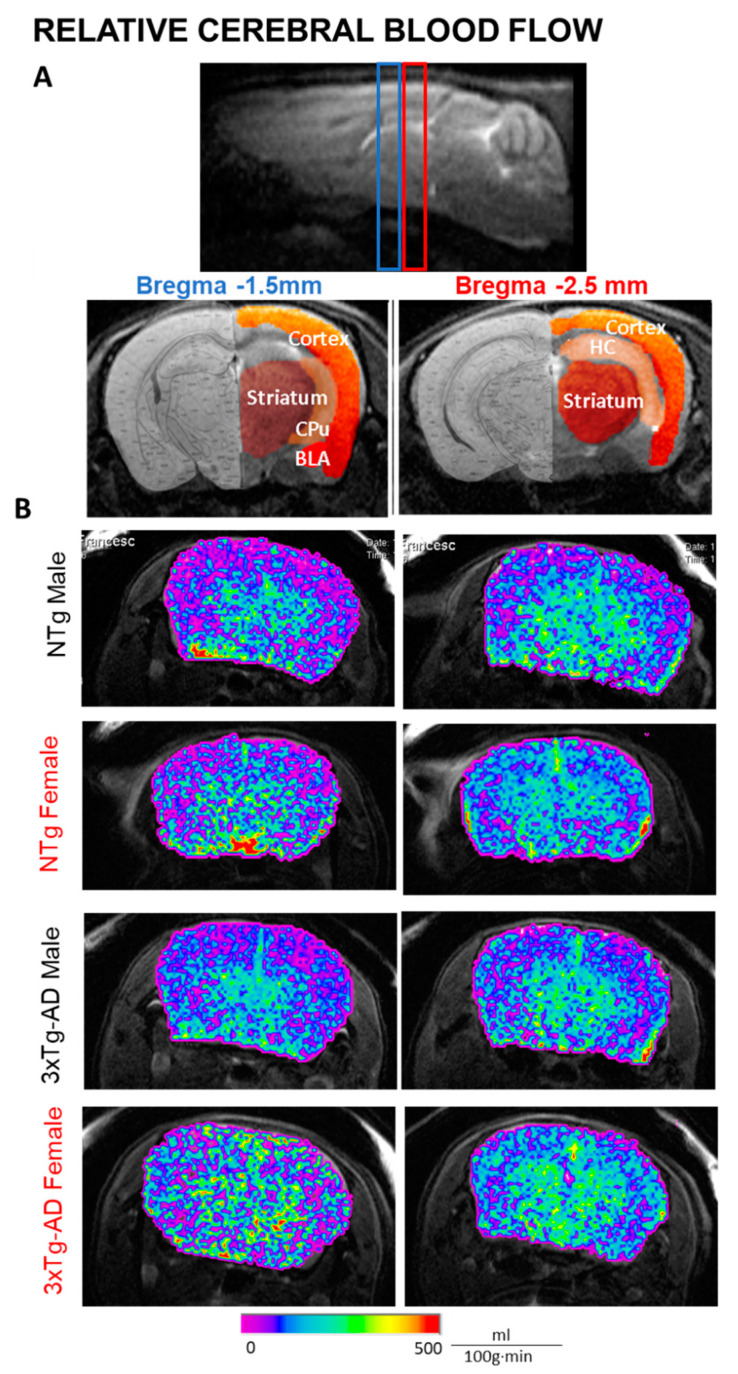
MRI arterial spin labeling (ASL)—relative cerebral blood flow in regions of interest. (A) Global cerebral blood flow and five regions of interest were examined in the present study from two different sections (Bregma −1.5 mm, −2.5 mm). Cortex; striatum; caudate putamen (CPu); basolateral amygdala (BLA); hippocampus (HC). (B) Representative images from rCBF maps superimposed to T2w-image from NTg male, NTg female, 3xTg-AD male, and 3xTg-AD female.
